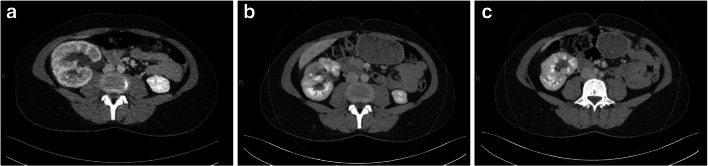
Contrast-enhanced CT scan. a At presentation. b, c At 4 months after discharge. There is an evident decrease in the volume of the right kidney compared to (a). Furthermore, notice the irregular aspect and decreased density of the surface of the interpolar region (b) and lower pole (c) at the site of the previous infection. This indicates scarring of the renal cortex. Multiple small concrements in the right kidney remain
